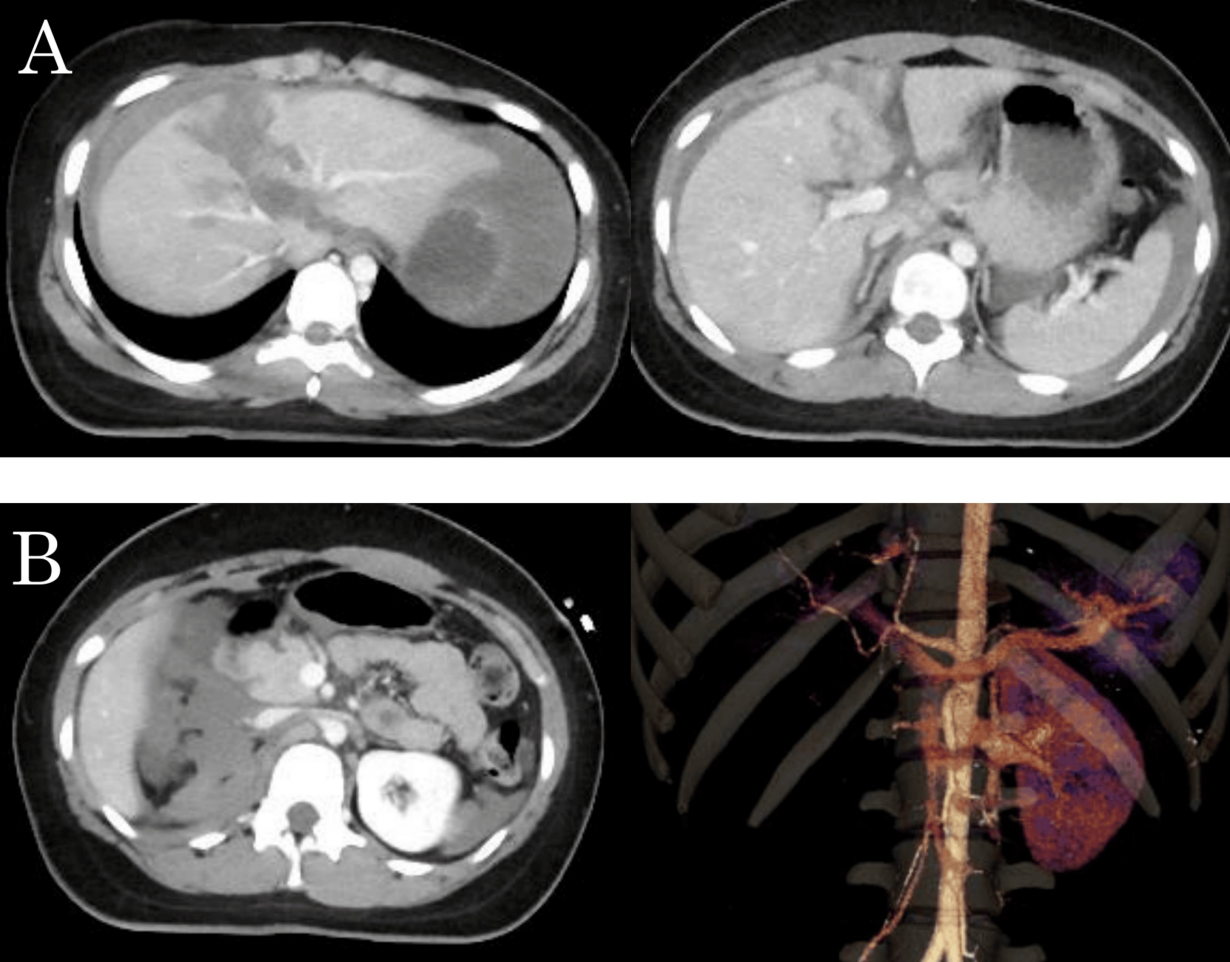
Findings on computed tomography upon arrival (A) Contrast-enhanced computed tomography showing a deep hepatic laceration extending from segment IV to V (AAST-OIS grade IV). (B) Right renal injury with infarction suspected to be a complete hilar avulsion (AAST-OIS grade V). AAST-OIS, American Association for the Surgery of Trauma Organ Injury Scale
